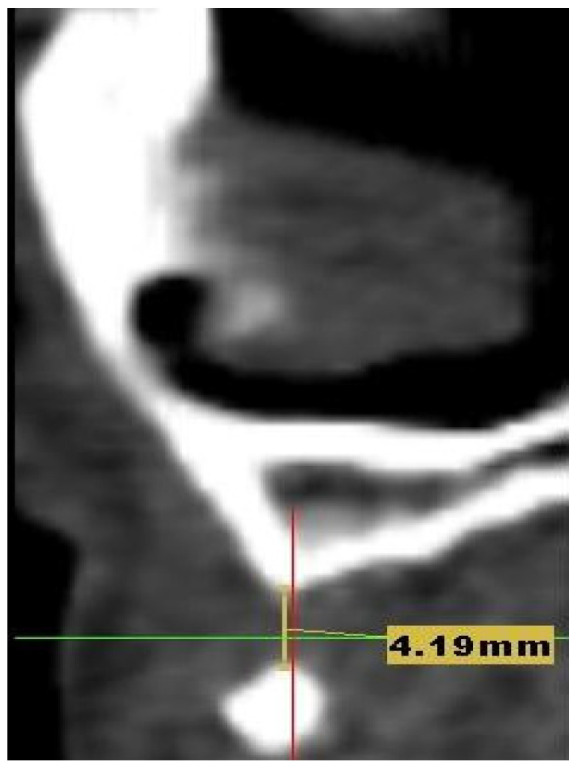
The CBCT aspect of the initial situation: the defect measures 4.19 mm in length and 4.2 mm in width of bone deficiency.
